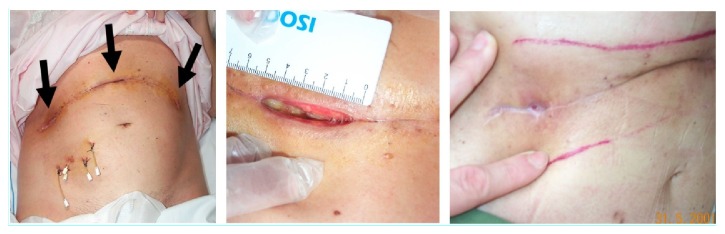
Delayed healing after a pancreatic cancer surgery. Fifty-three-year-old female patient with pancreatic carcinoma. During 1st surgery, tumor resection was not possible. She was treated with RT and CT. During a 2nd surgical intervention, complete tumor resection was not possible due to large vessel infiltration, and catheters were inserted for brachytherapy (a localized way for RT administration). (*Left*) Fourteen days after surgery. Note the catheters for brachytherapy in the lower-right abdomen. At this time, there were 3 wounds indicating delayed healing (arrows), all of which are larger than 40x10x10 mm. Pancreatic cancer cells were confirmed in the wounds. Local O_3_T was applied together with a 3rd course of RT (2nd external beam RT—this time, with electrons). (*Right*) Six weeks later. Complete wound healing (despite tumor cells) was observed during the 3rd RT after 15 sessions of local O_3_T.
